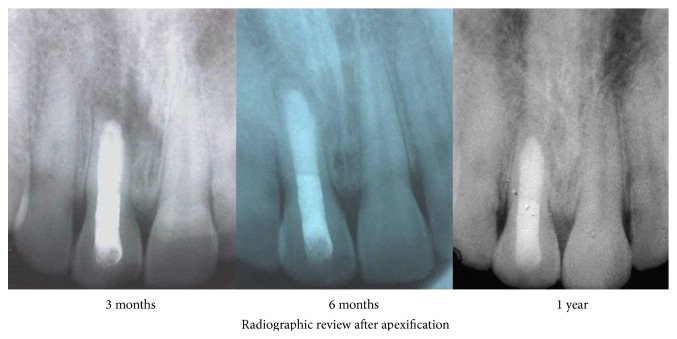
Postoperative radiographs after apexification.
